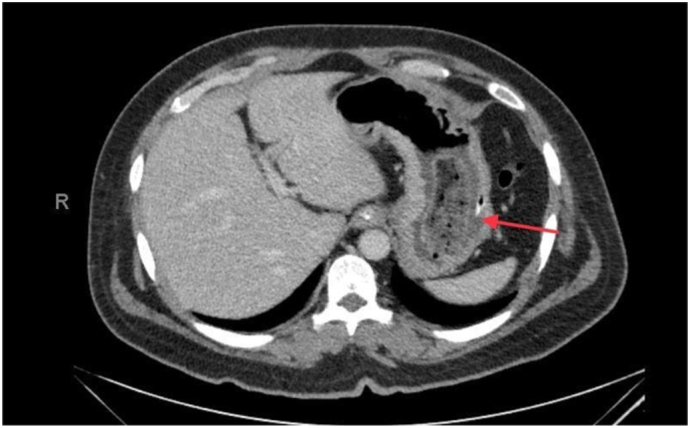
A axial plane of CT abdomen showing a large intra-gastric mass, pointed by the red arrow.
